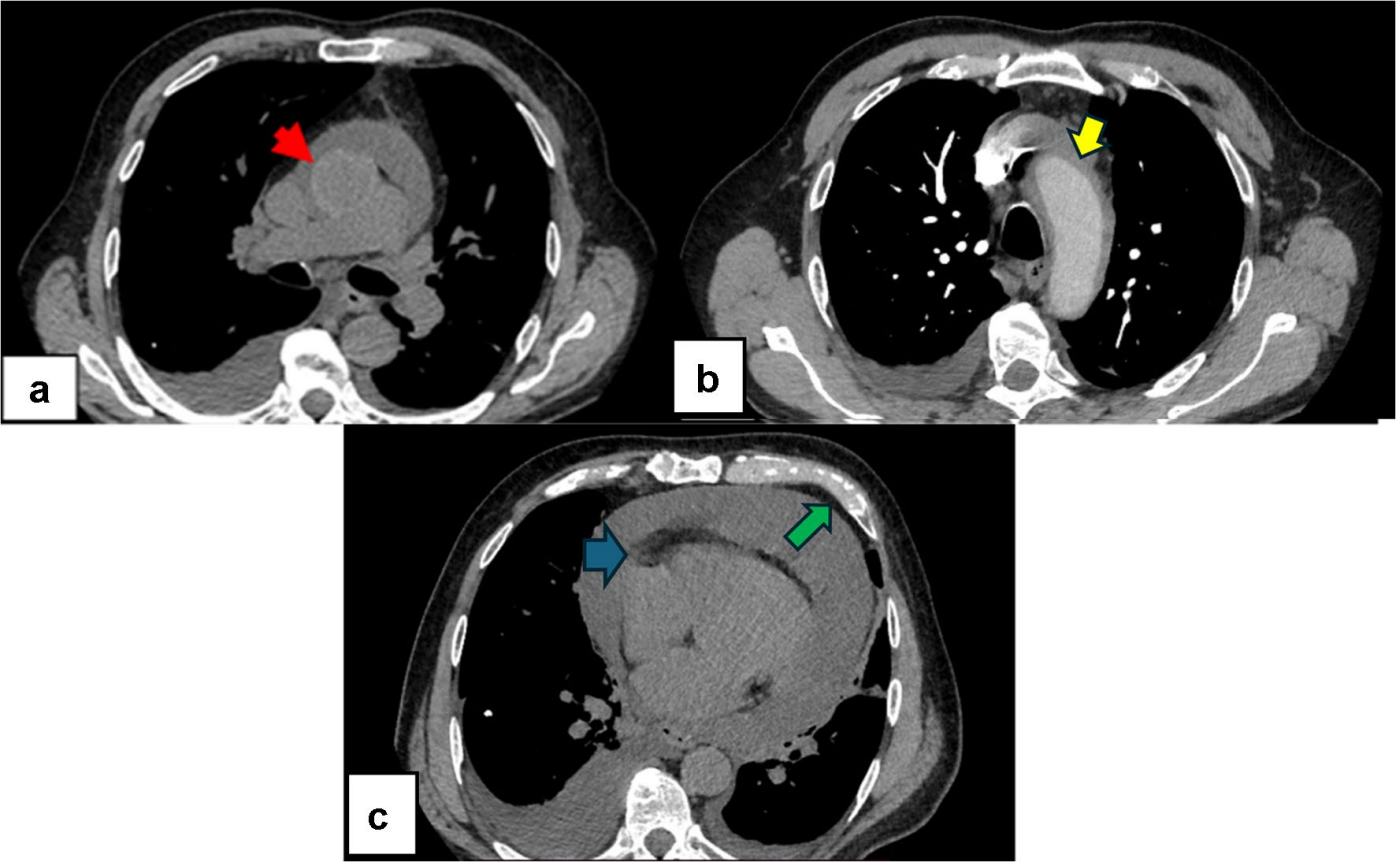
a Computed tomography pulmonary angiography (CTPA) scan showcasing the abnormal thickening of the ascending aortic wall, suggesting the presence of intramural hematoma (red arrow). b Computed tomography pulmonary angiography (CTPA) scan showcasing extent of the supposed intramural hematoma distal to the origin of the left subclavian artery (yellow arrow). c Computed tomography pulmonary angiography (CTPA) scan showcasing pericardial effusion (blue arrow) and pleural effusion (green arrow)
